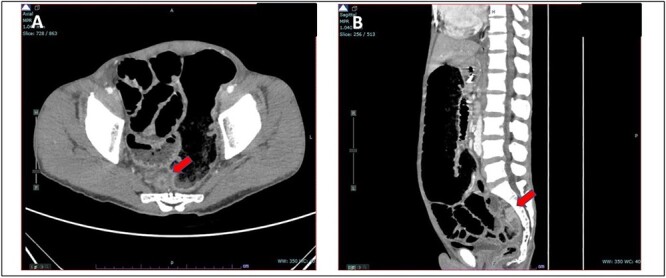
(A) Axial view and (B) sagittal view of computed tomography showed short segment circumferential enhancing bowel wall thickening at rectosigmoid region causing intraluminal obstruction (red arrow).
